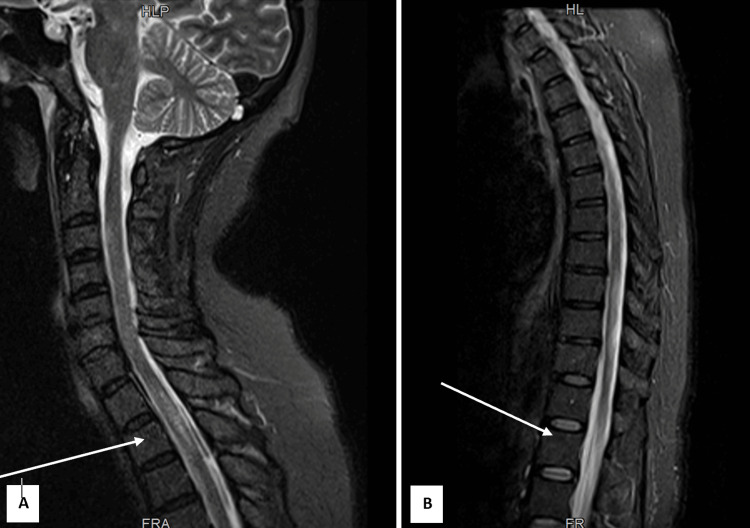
Sagittal magnetic resonance imaging scans at admission. The images (A) cervicodorsal region and (B) thoracolumbar region show a T2 hyperintense signal extending from T4 to the conus medullaris, predominantly central in distribution (indicated by arrows with greater intensity).
